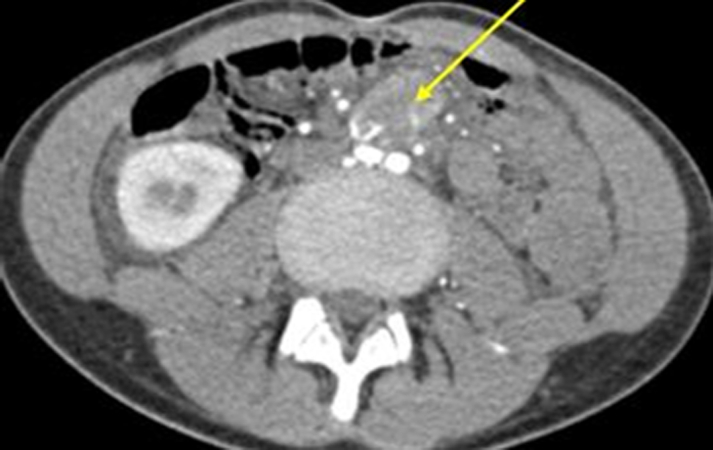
Axial Computed Tomography Angiography of the Abdomen and Pelvis Showed a Hyperenhancing Mass at the Aortic Bifurcation With Early Arterial Enhancement, Highly Suspicious for Being Malignant The yellow arrow indicates the hyperenhancing mass.
